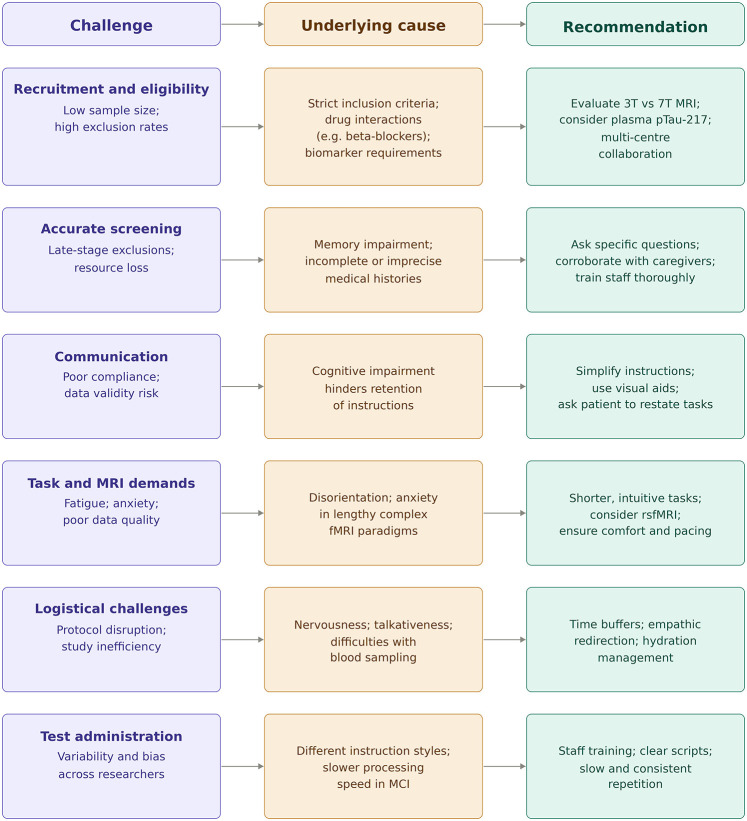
Shows the main challenges of a pharmacological 7T MRI study with AD population, underlying causes and recommendations. This is an ongoing study.
